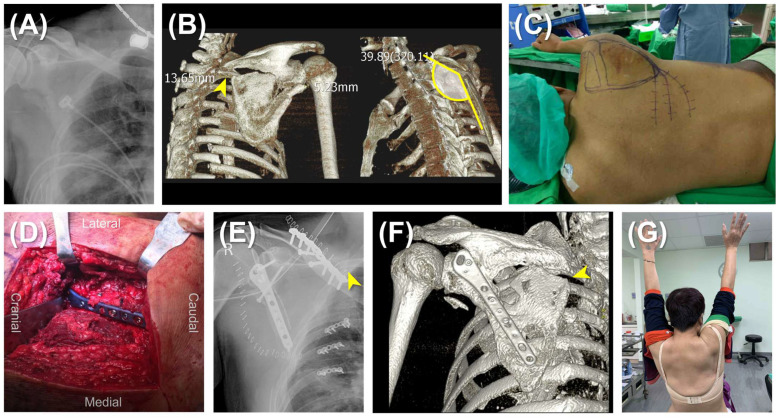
Single lateral plating for scapular fracture. (A) Preoperative imaging of a 58-year-old woman with a right scapular fracture (AO/OTA 14B(lm)) accompanied by ipsilateral clavicle and multiple rib fractures, hemothorax, and pneumothorax. (B) CT reconstruction demonstrating a marked displacement and 40° angulation of the scapular body at its lateral and medial borders (yellow arrowhead). (C) Patient positioned in the left lateral decubitus position for surgical stabilization. (D) Intraoperative view via the mirror Judet approach showing fixation of the scapular fracture with a single lateral anatomical locking plate and concurrent reduction and stabilization of multiple rib fractures. (E) Postoperative imaging revealing a persistent medial gap and resulting malunion (yellow arrowhead). (F) Another case of scapular body fracture. At 12-month follow-up, CT confirms solid osteosynthesis of the lateral border but malunion of the medial border persists (yellow arrowhead). (G) Clinical photograph of the 58-year-old woman at 12 months demonstrating restoration of full active shoulder abduction.
